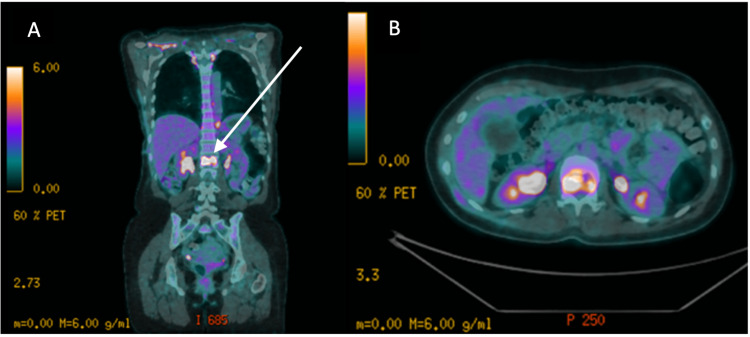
Positron emission tomography-computed tomography findings. Scan showing metabolically avid lesion within the vertebral body L1 concordant with the report of the plain computed tomography. There were no other soft-tissue or bony metastases revealed apart from the aforementioned small iliac crest deposit. A: coronal section; B: axial cut at the level of L1.
